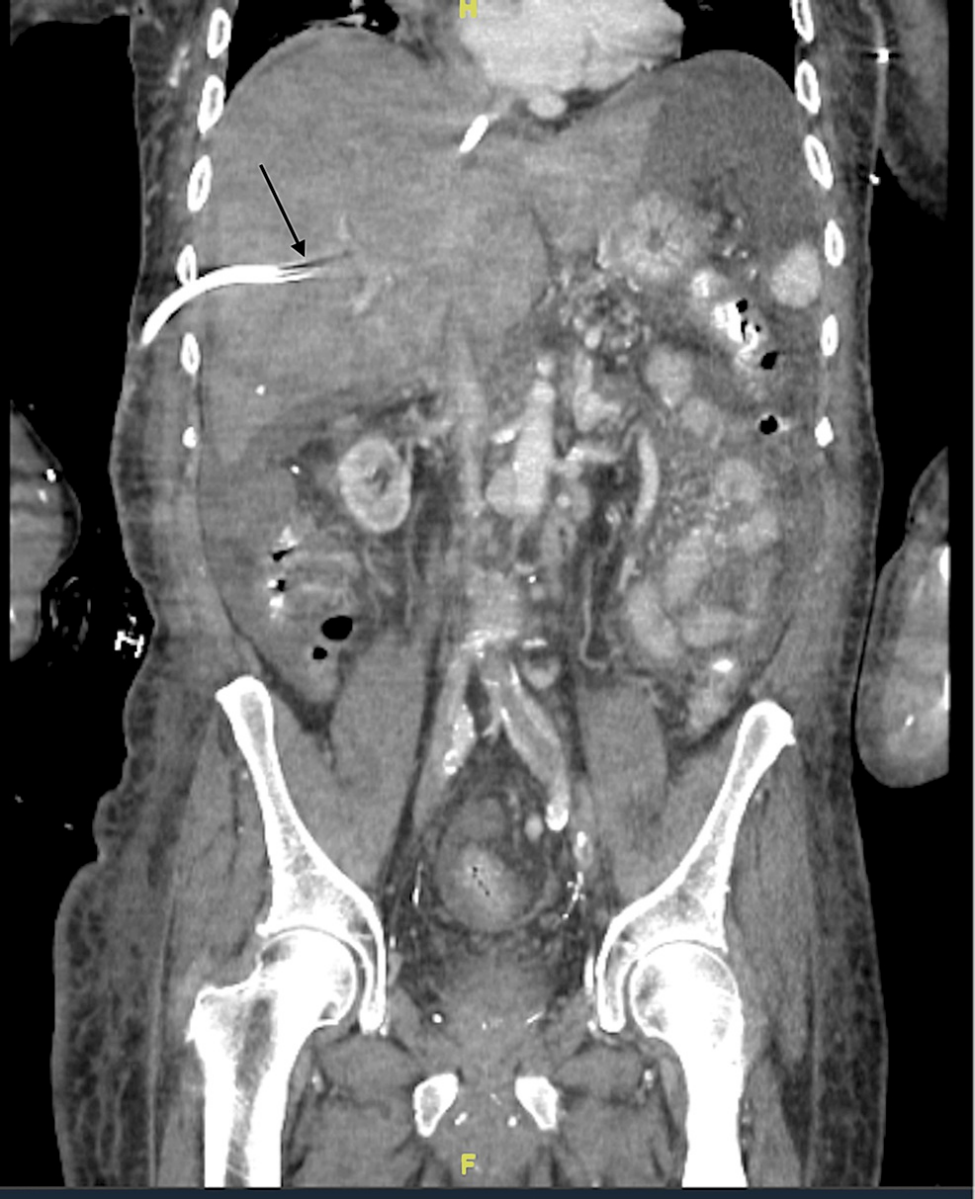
Triple-phase CT of the abdomen The image shows a diffusely enlarged and edematous liver with a lack of opacification of the hepatic veins, suggestive of hepatic vein thrombosis. Transhepatic catheter with near complete occlusion of flow, as shown by the arrow CT: computed tomography
